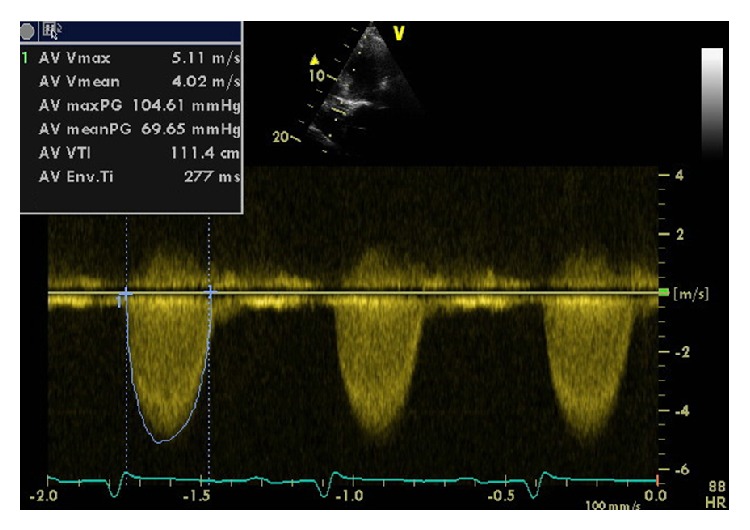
Echocardiography, continuous-wave spectral Doppler across the aortic valve showed severe aortic stenosis with mean gradient 70 mmHg and peak gradient 105 mmHg.
